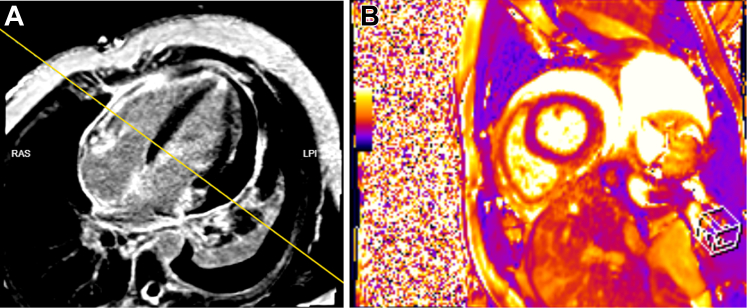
Cardiac MRI at Initial Presentation Cardiac MRI with contrast T2 phase showing mid global LV and RV hypokinesia with asynchronous LV contraction and mild hypokinesia of the midventricular anteroseptal segment. The LV ejection fraction was 45%, and the RV ejection fraction was 43%. An ill-defined LGE in the basal inferior and inferolateral segment with myocardial edema affecting these segments was suggestive of myopericarditis. Moderate pericardial effusion can also be seen. LGE = late gadolinium enhancement; LV = left ventricle; MRI = magnetic resonance imaging; RV = right ventricle.
